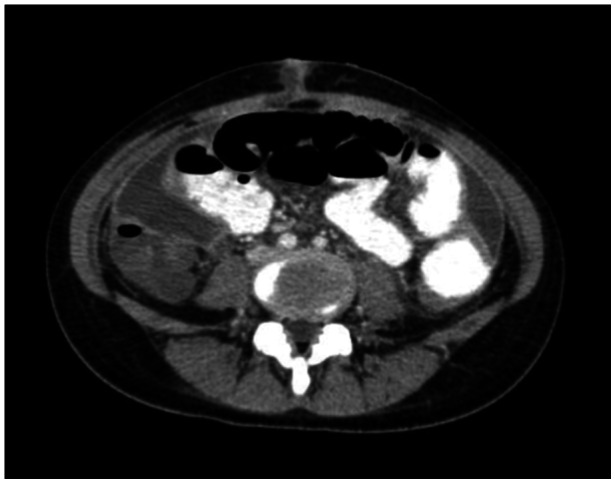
Increased smooth thickening of the peritoneum in CT scan. CT, computed tomography.
